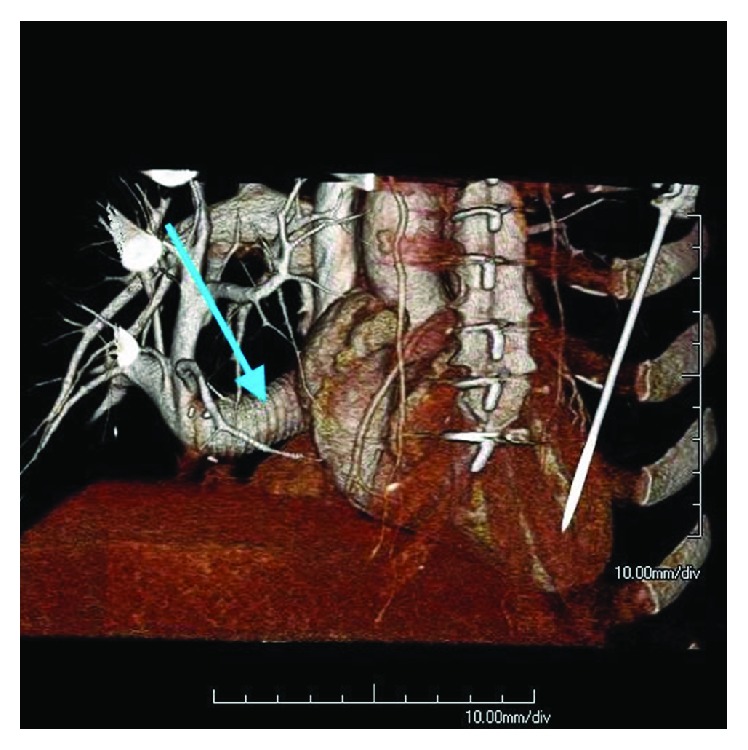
3D volume rendering of postoperative CTA from a less oblique RAO view. Arrow pointing to the artificial baffle diverting the scimitar vein toward the left atrium behind the right atrium.
